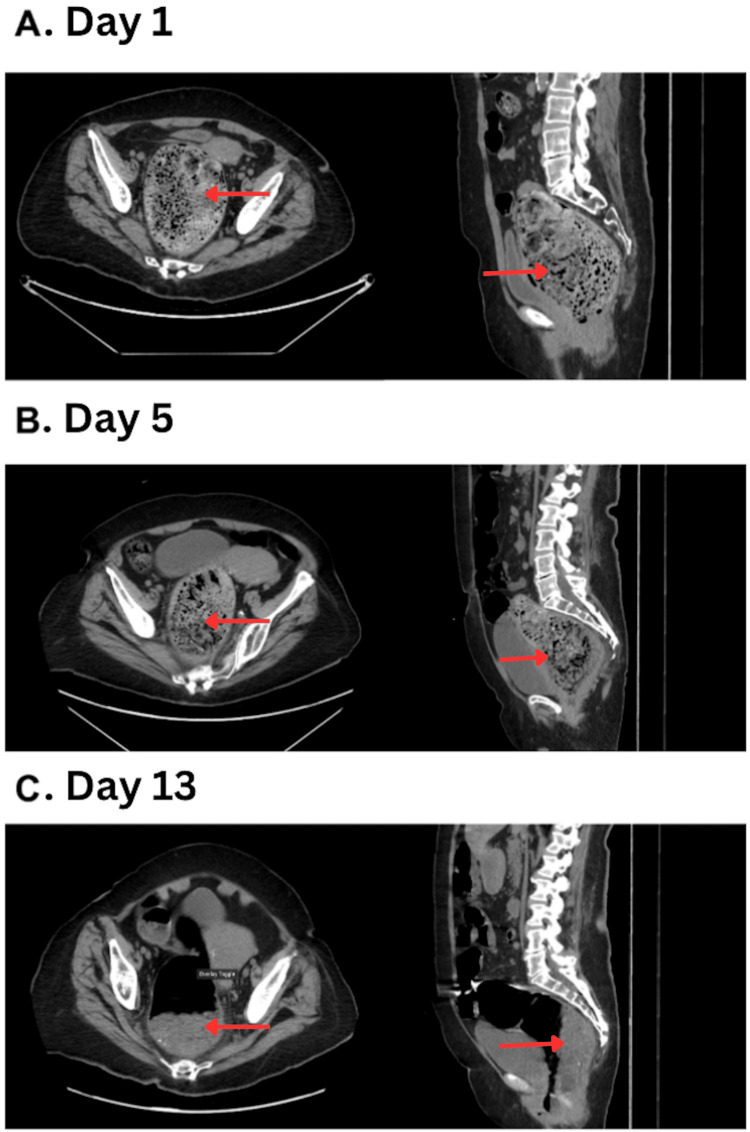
CT imaging of the patient during hospitalization. Panel A demonstrates the significant fecal impaction on admission. Panel B indicates fecaloma persistence after conservative measures on hospital day 5. Panel C shows significant reduction in size of the fecaloma one day after administration of a classic Coca-Cola enema. The red arrows indicate stool burden over the hospital course.
